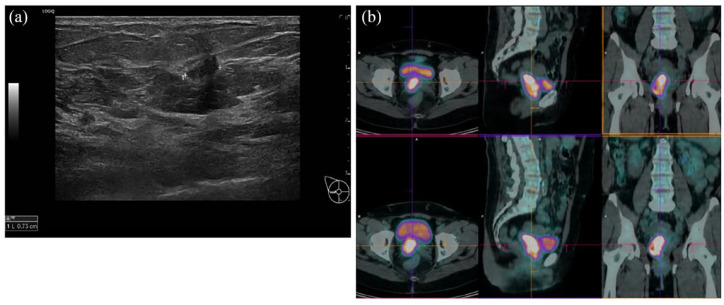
(a) Breast ultrasound: heterogeneous background echotexture with a hypoechoic area of 7 mm diameter. (b) PET-CT with FDG, performed for the initial cancer staging on April 2022, identified a hypermetabolic pathological tissue of 35 × 58 cm^2^ on the right side of the cervix, with extension to the vagina and the right parametrium. FDG, fluorodeoxyglucose; PET-CT, positron emission tomography/computed tomography.
